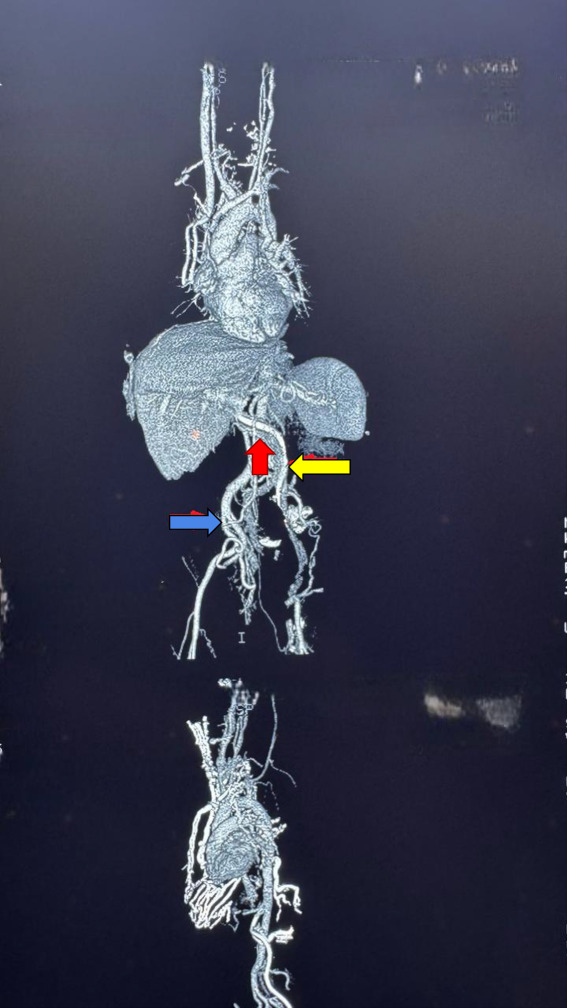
3D CT angiographic reconstruction demonstrates duplication of the IVC, with two parallel ascending venous pathways originating from the lower extremities. These vessels converge at the level of the renal veins—highlighted by the red arrow—forming a single IVC above this point. The yellow arrow indicates the left-sided IVC and the intravascular trajectory of the catheter. By contrast, the blue arrow points to the right-sided IVC, which shows a markedly reduced caliber compared with the contralateral vein, consistent with significant stenosis. In addition, there is evidence of severe stenosis of the SVC, characterized by pronounced narrowing of its lumen in the upper thoracic segment. Associated collateral circulation is visible, suggestive of compensatory venous hypertension. SVC, superior vena cava.
